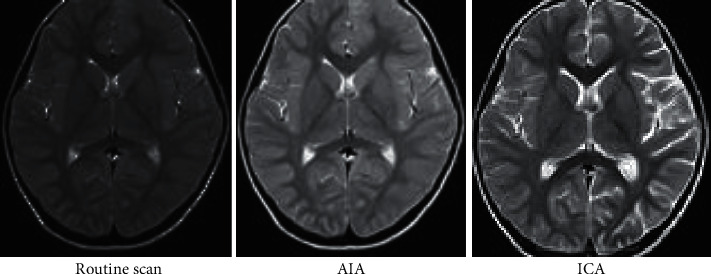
Comparison on the enhancement effect of algorithms on MRI images.
